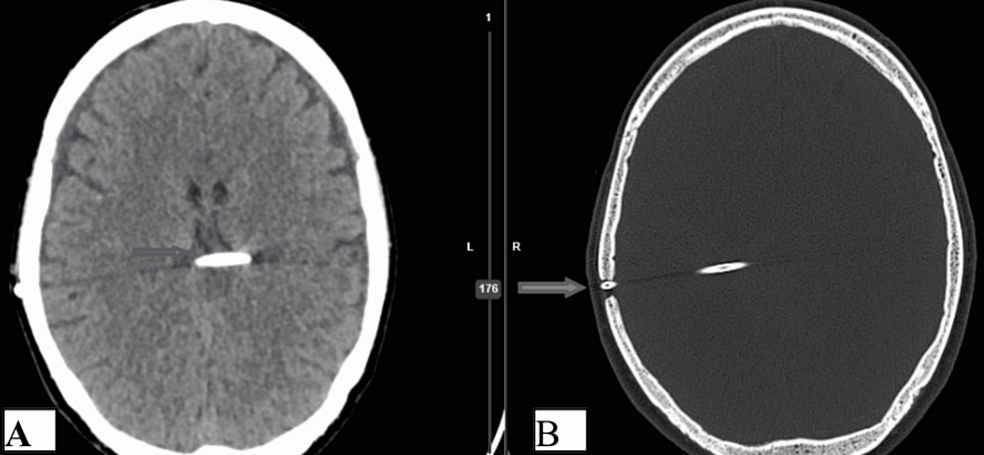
Postoperative CT brain plane study (axial section) and CT bone window of the same patient showing the burr hole defect in right parietal bone, VP shunt in situ with its tip in the trigone of the lateral ventricle.
